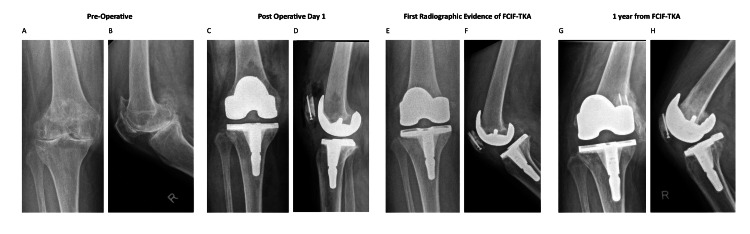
Patient 6 AP and lateral knee FCIF-TKA radiographs A: preoperative AP film, B: preoperative lateral film, C: postoperative day 1 AP film, D: postoperative day 1 lateral film, E: first radiographic evidence of FCIF-TKA AP film, F: first radiographic evidence of FCIF-TKA lateral film, G: one year from FCIF-TKA AP film, H: one year from FCIF-TKA lateral film AP: anterior-posterior, FCIF-TKA: femoral condyle insufficiency fractures following total knee arthroplasty
